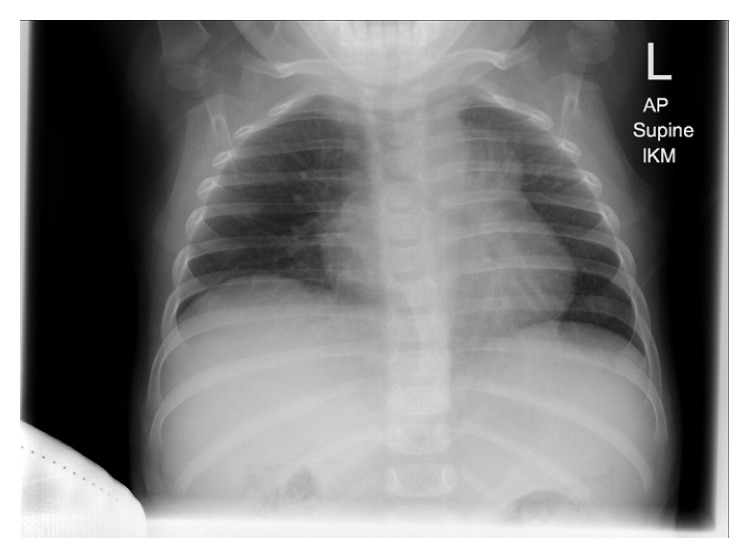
X-ray of chest showing resolution of the previously seen mediastinal mass, except for a small left parahilar opacity.
